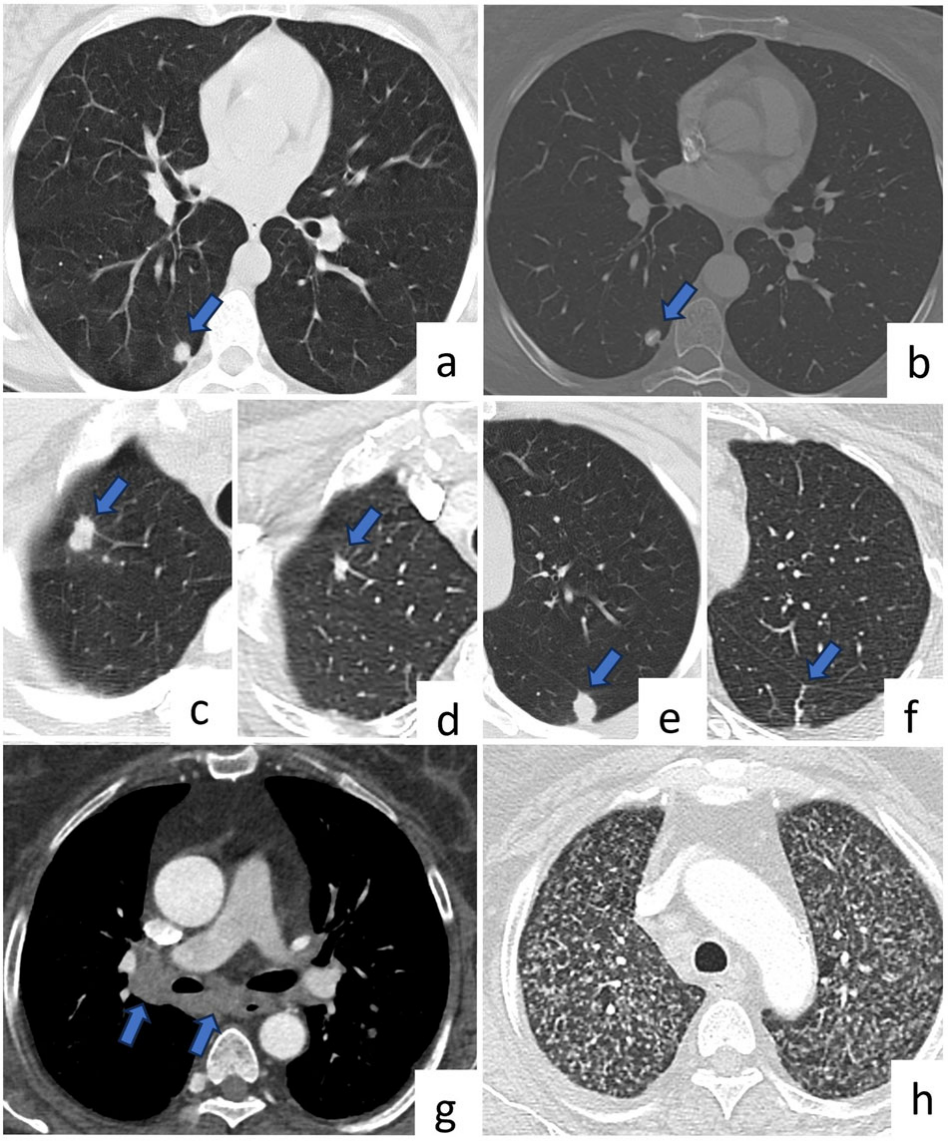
Tuberculosis. A 57-year-old patient with diabetes has multiple lung nodules incidentally detected. Axial CT images (a–f) show incidentally detected multiple lung nodules (arrows) diagnosed as pulmonary tuberculosis. On axial CT image (bone window) (b), a calcified nodule is seen. Axial CT images obtained between 2006 (c, e) and 2022 (d, f) show that there is a partial size reduction of the nodule in the right upper lobe (c, d), and the nodule in the left lower lobe has turned into a fibrotic band (e, f) after antituberculosis treatment. In a 71-year-old female patient diagnosed with miliary tuberculosis, axial CT image reveals right hilar and subcarinal lymphadenopathy (g, arrows), along with widespread and numerous micronodules in both lungs (h)
